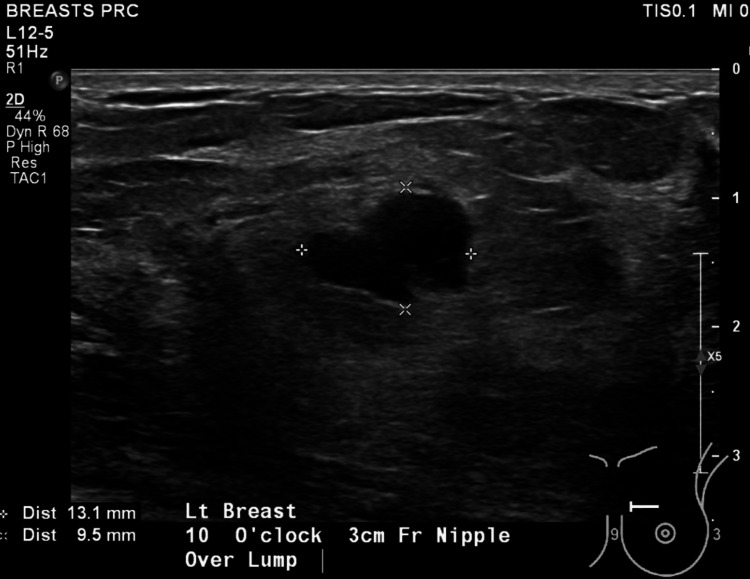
Ultrasound of her right breast on her second presentation.
